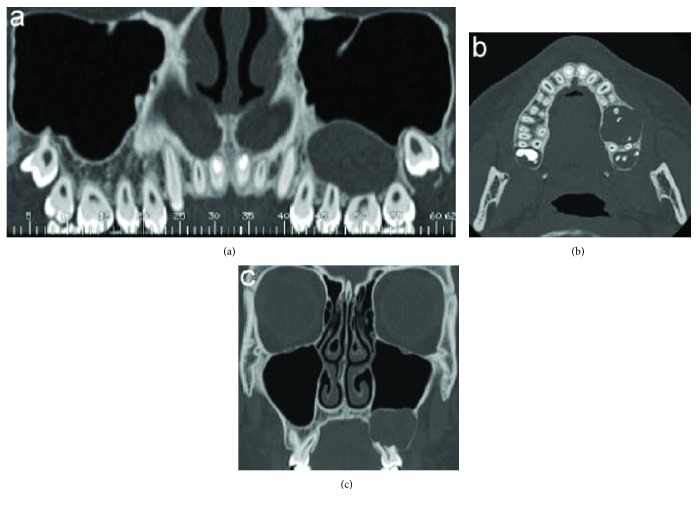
Computed tomography scan: (a) panorex view: unilocular, well defined, hypodense area extending from the mesial root of 2.4 up to the distal root of 2.7 and in the apicocoronal direction from the sinus floor to the alveolar ridge; (b) axial view: limited expansion and thinning of the buccal and palatal cortical plates, erosion of the buccal wall, and some small foci of radiopacity; and (c) coronal view: root resorption of the first molar.
